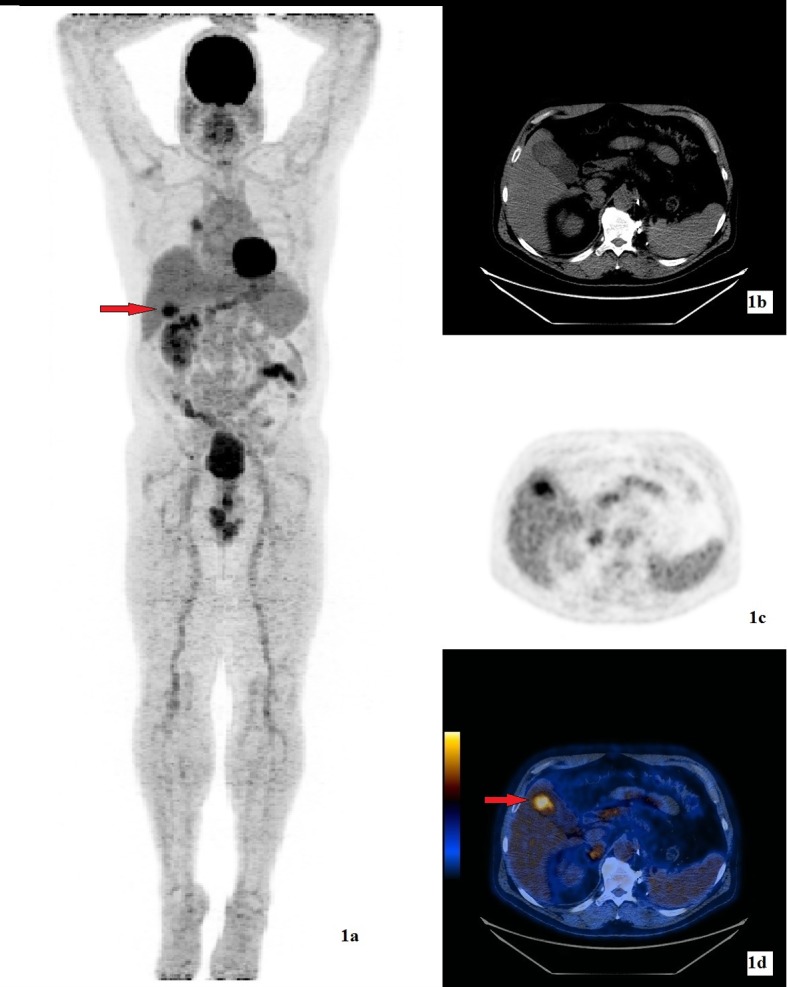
(a) 3D MIP image of FDG-PET/CT study showing a hypermetabolic lesion (arrow) in the gallbladder. (b) CT showing a non-specific hyperdense nodule within the gallbladder. The gallbladder was otherwise normal with no wall thickening and no pericholecystic fluid. (c) FDG emission image. (d) Hybrid FDG-PET/CT image showing a hypermetabolic soft tissue density of SUVmax 6 (arrow) within the fundus of the gallbladder.
